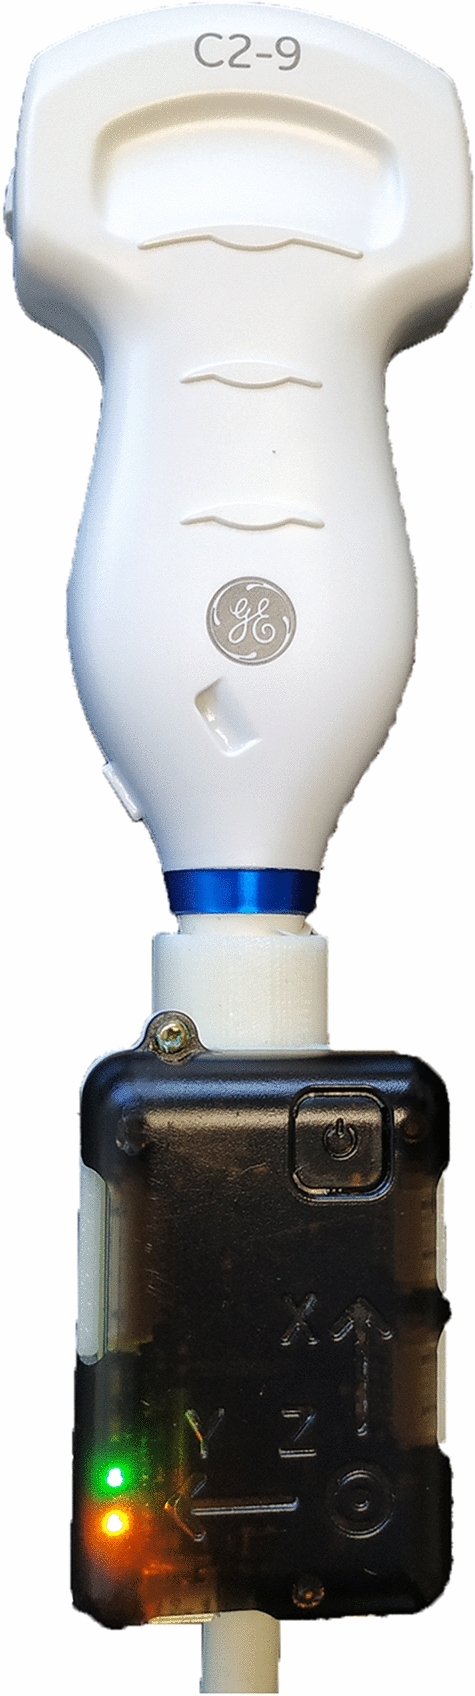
Inertial Measurement Unit (IMU) device attached to a curvilinear ultrasound transducer, acting as the sonographer hand tracking device. Figure created using Microsoft Office version 365 (https://office.microsoft.com/).
